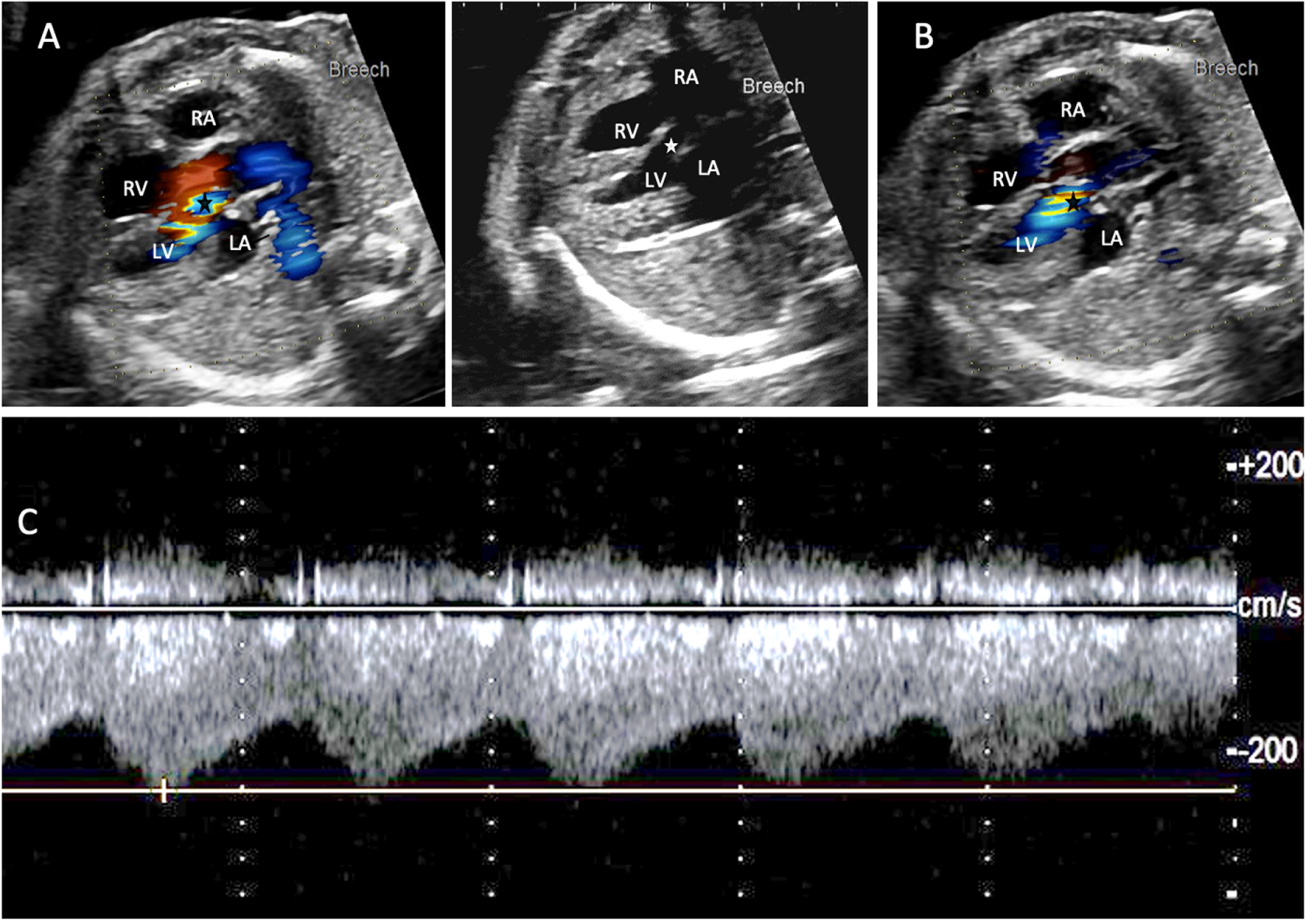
Fetal echocardiographic four-chamber view showing the Aortic-left atrial tunnel in both a systole and b diastole c Pulse wave doppler waveform obtained at the site of the aortic-left atrial tunnel (star) demonstrates a high velocity continuous waveform pattern diagnostic of this lesion. *RA* right atrium, *RV* right ventricle, *LV* left ventricle, *LA* left atrium
